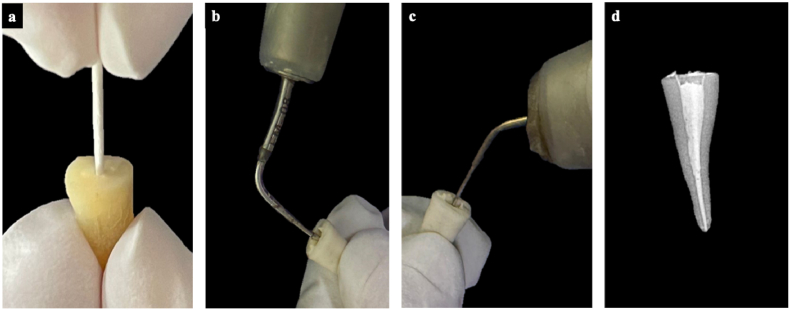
Representative steps of the obturation procedure using warm vertical compaction technique. (a) Master gutta-percha cone coated with sealer and positioned to working length, (b) Down-pack phase using System B heat source for vertical compaction of gutta-percha, (c) Backfilling of the canal with thermoplasticized gutta-percha to achieve a three-dimensional seal, and (d) Post-obturation radiograph demonstrating the final quality of root canal filling.
